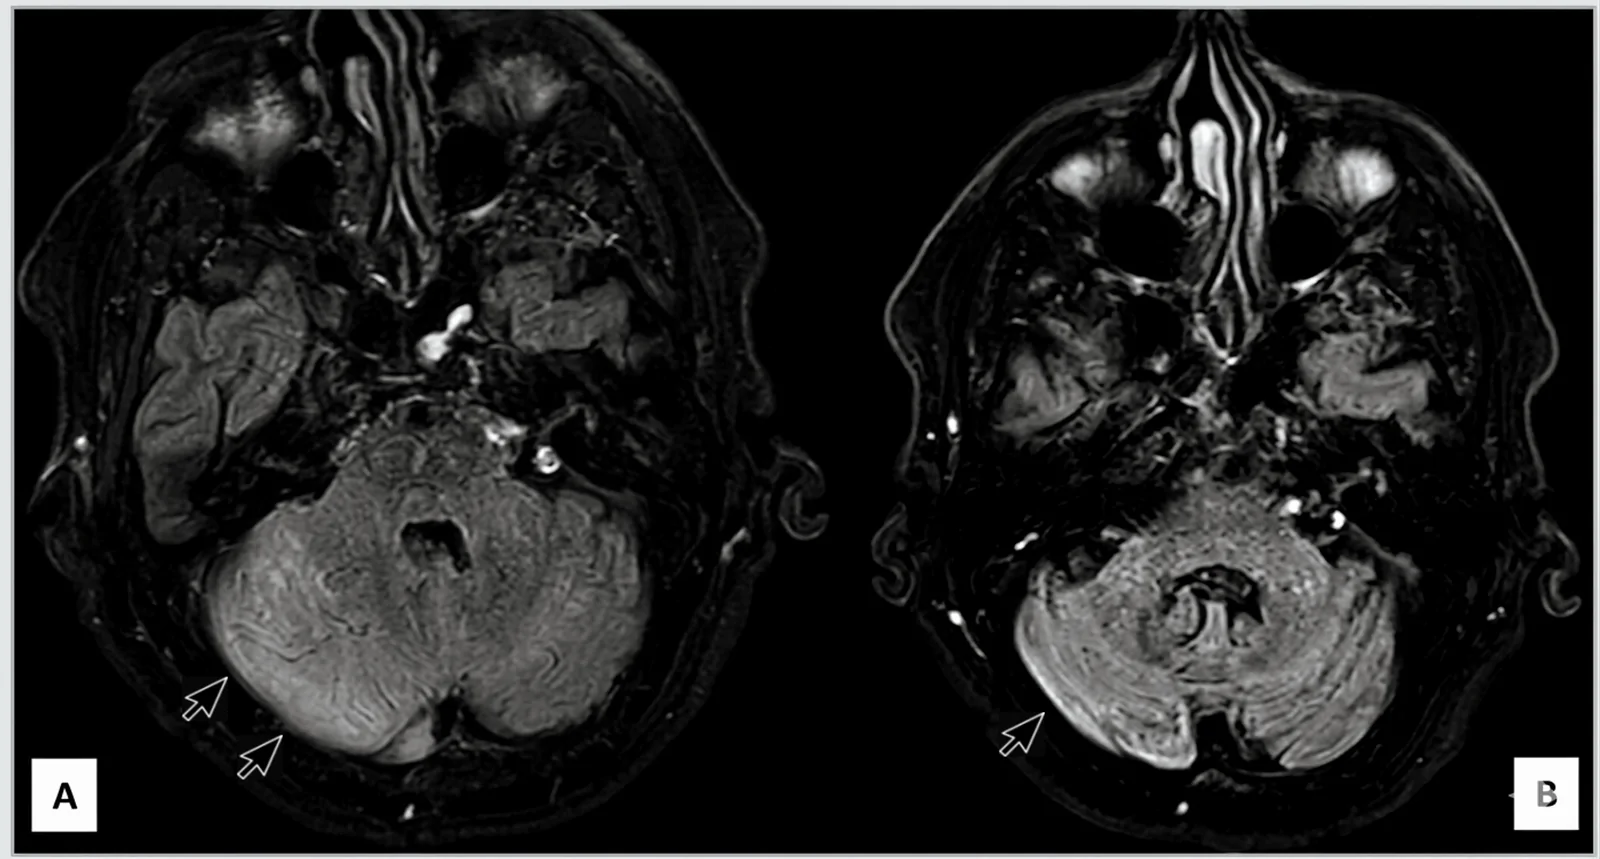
T2-FLAIR brain MRI at admission and five-month follow-up. (A) T2-FLAIR brain MRI obtained at hospital admission with blurred FLAIR hypersignal and slight T2 hypersignal in the right cerebellar hemispheric cortico-subcortical region, compatible with vasogenic edema. (B) T2-FLAIR brain MRI performed during follow-up, carried out after five months, showed a gliotic lesion in the right cerebellar hemisphere. The arrowheads indicate the area of vasogenic edema on the baseline MRI (A) and the residual gliotic lesion on the five-month follow-up MRI (B). FLAIR: fluid-attenuated inversion recovery
